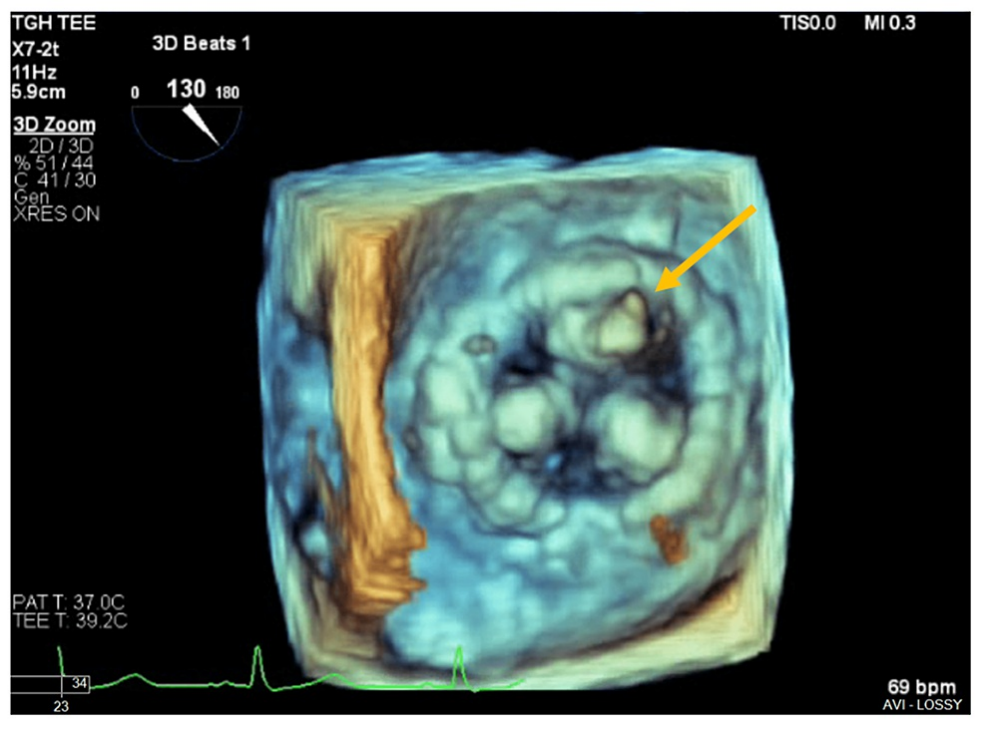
Three-dimensional TEE of the bioprosthetic mitral valve (surgeon’s view), without evidence of vegetation, thrombus, or pannus. The flail anterior leaflet is visible (yellow arrow). TEE: Transesophageal echocardiogram
